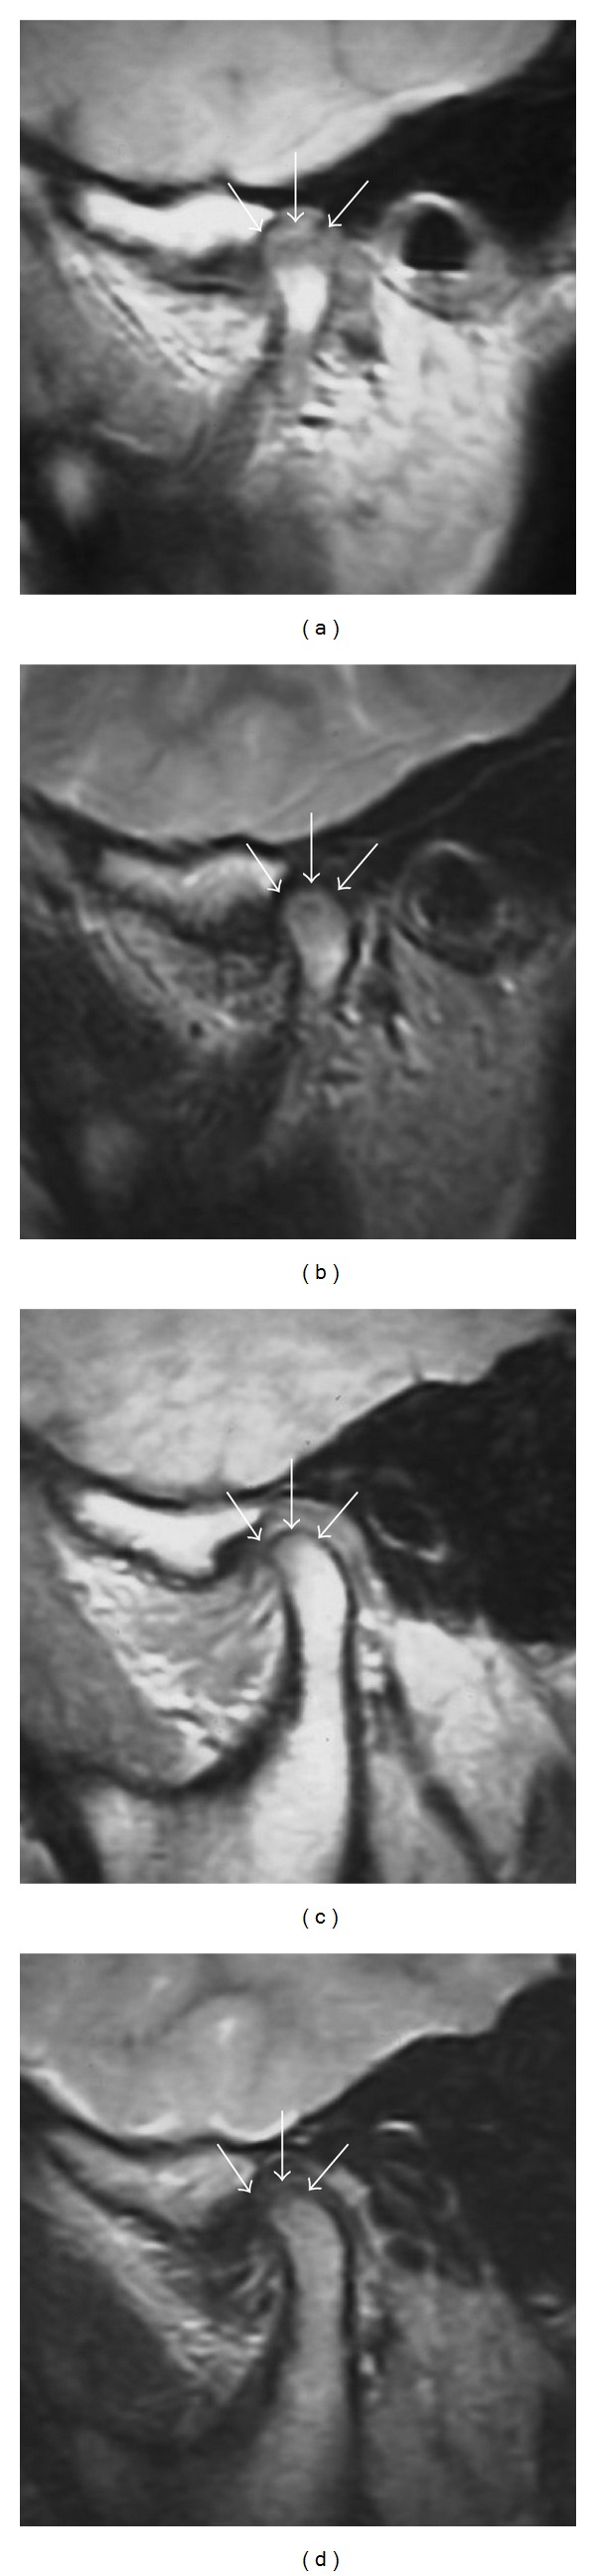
Alteration of mandibular condyles on MRI in growing schoolchildren over time. (a) DCLSs on the top of the mandibular condyles (arrows) appeared as high-intensity stripes over a low-intensity area on fast spin-echo intermediate-weighted images of a healthy 12-year-old boy at the first examination. The voided signal line at the top of the right mandibular condyle was not seen. The imaging parameters used in the MR sequences included 1050 ms TR, 15 ms effective echo time, flip angle of 90°, a 15 × 15 cm field of view, a matrix of 160 × 288 pixels, 17 mm slab thickness, and 3 mm section thickness. (b) High-intensity stripes (arrows) were found to coincide with DCLSs on the top of the mandibular condyles; this was found using fast spin-echo T2-weighted images of the same subject as Figure 1(a). The imaging parameters used in the MR sequences included 3500 ms TR, 108 ms effective echo time, flip angle of 90°, a 15 × 15 cm field of view, a matrix of 160 × 288 pixels, 17 mm slab thickness, and 3 mm section thickness. (c) DCLS at the top of the left mandibular condyle, apparent at the first examination, had disappeared by the second examination three years later in the same subjects as Figures 1(a) and 1(b). The imaging parameters used in the MR sequences included 1050 ms TR, 15 ms effective echo time, flip angle of 90°, a 15 × 15 cm field of view, a matrix of 160 × 288 pixels, 17 mm slab thickness, and 3 mm section thickness. (d) High-intensity stripes (arrows) were not seen at the top of the mandibular condyles at the second examination three years after the first one in the same subjects as Figures 1(a), 1(b), and 2(a). The imaging parameters used in the MR sequences included 3500 ms TR, 108 ms effective echo time, flip angle of 90°, a 15 × 15 cm field of view, a matrix of 160 × 288 pixels, 17 mm slab thickness, and 3 mm section thickness.
